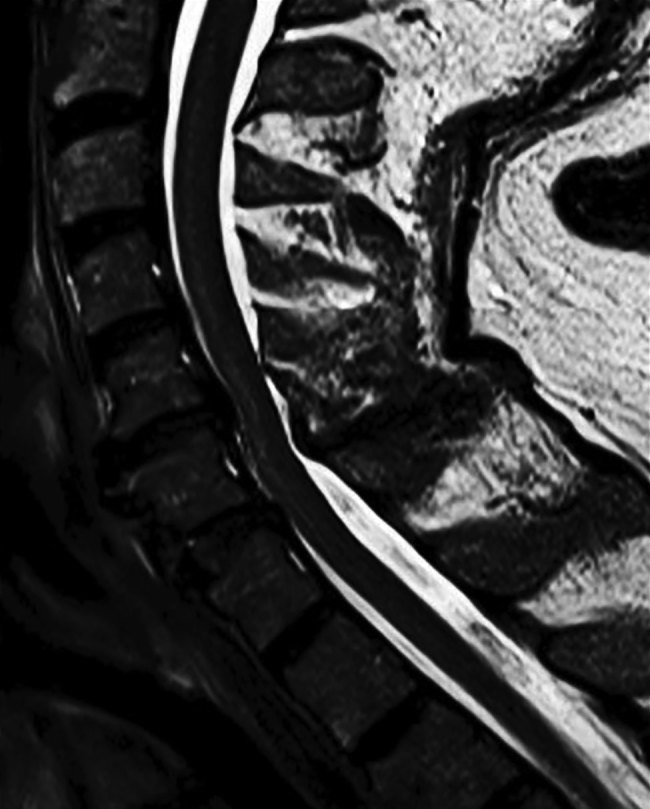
Sagittal T2-weighted image of cervical spinal cord in a patient with Powassan virus infection, Canada. A longitudinal hyperintensity of the anterior horn is visible from C3 to C6.
